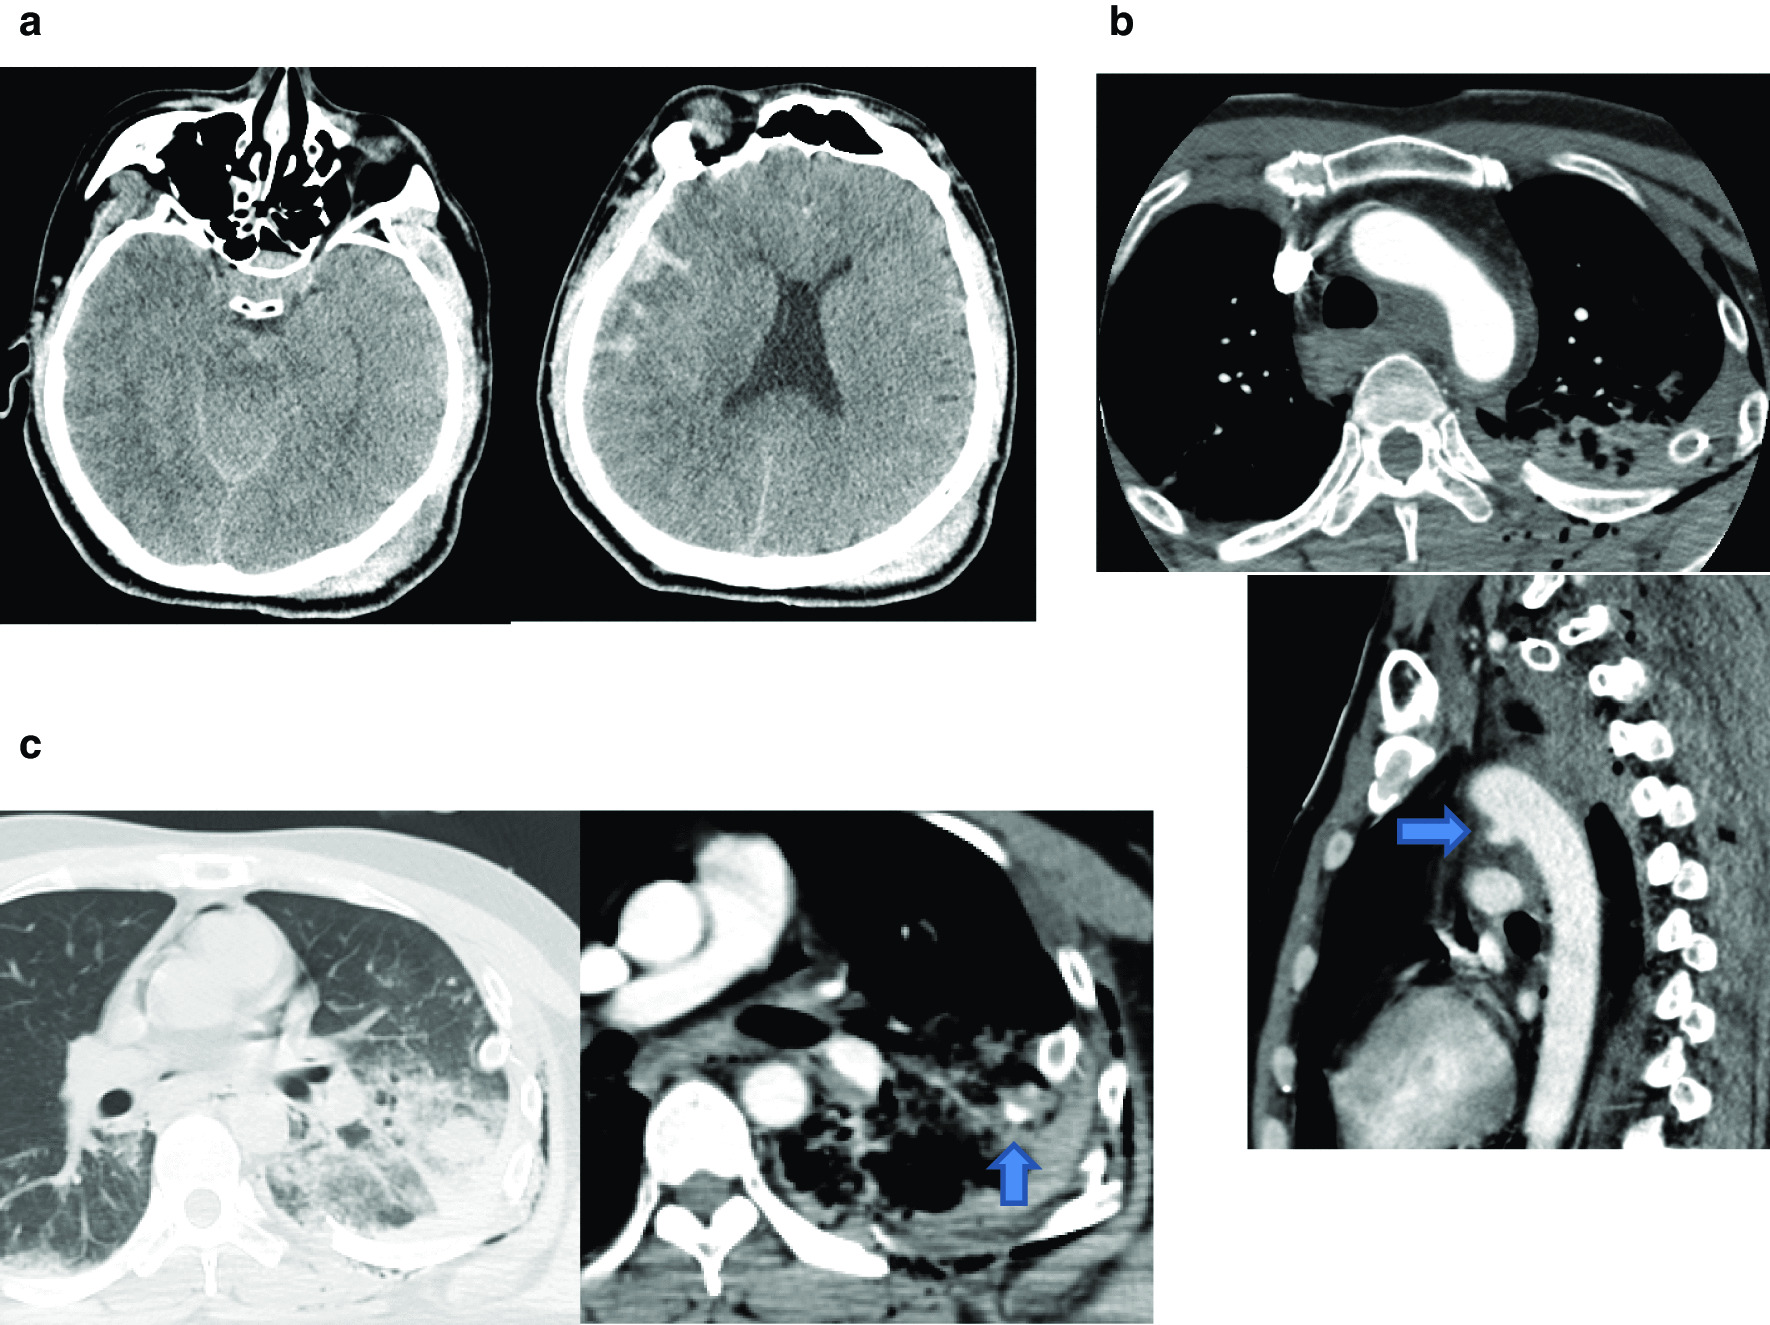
Cranial and thoracic CT images. a Right SDH and SAH with brain swelling. b Thoracic aorta injury with pseudoaneurysm indicated by the blue arrow. c Left lung contusion with contrast agent leakage indicated by the blue arrow
